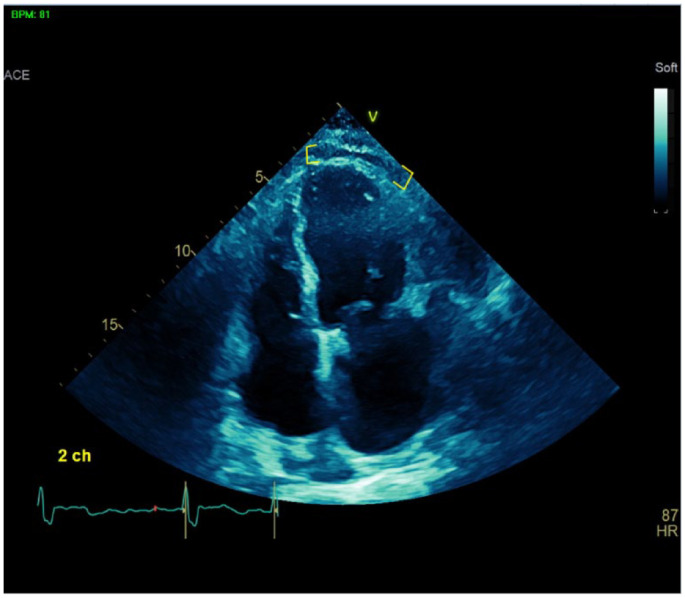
Transthoracic echocardiogram of pericardial effusion (yellow brackets) postremoval of left atrial myxoma.
